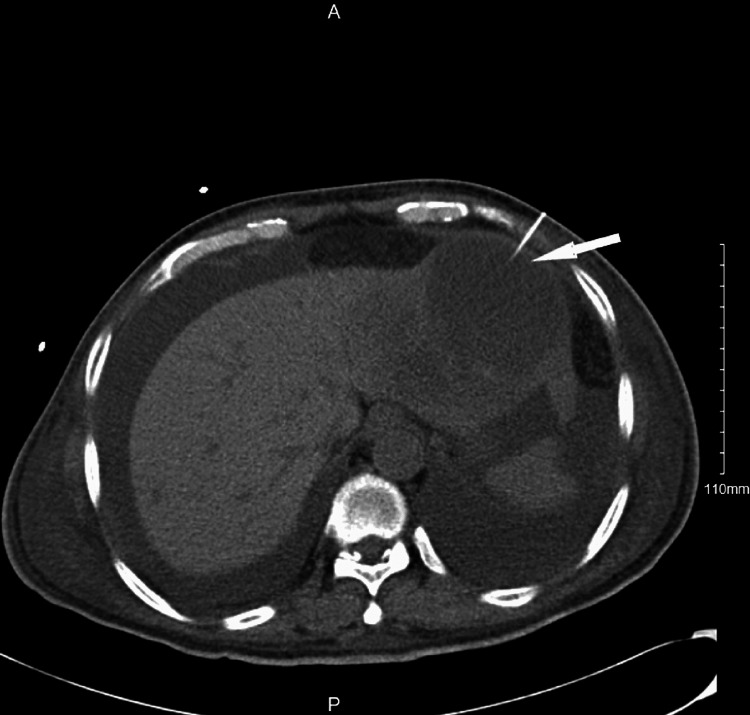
Computed tomography (CT) guided aspiration of hepatic cyst (arrow).
